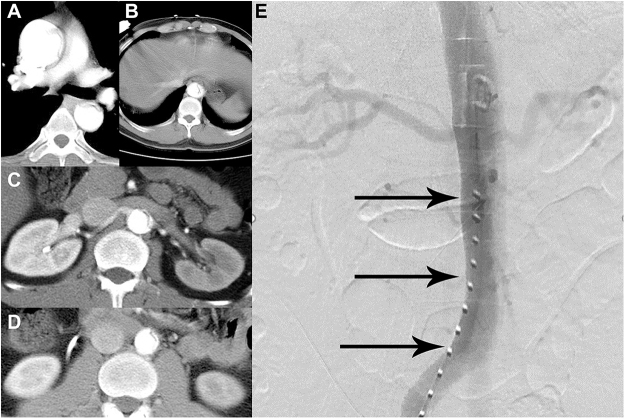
Accidental entry into the FL occurred at the femoral puncture site because the FL-perfused iliac artery was selected by a clinician at our institution. Preoperative CT ((A) ascending aorta, (B) hepatic, (C) pararenal, and (D) infrarenal levels) showing the TL extending along the left anterior side below the renal arteries. Intraoperative angiography (E) revealed that the guidewire had initially entered the FL.
